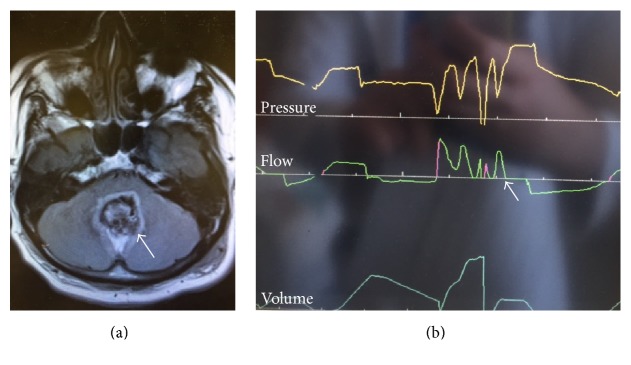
Magnetic resonance imaging of the brain. (a) T2 fluid attenuated inversion recovery (FLAIR) sequence showing medulloblastoma status after resection causing effacement of the fourth ventricle (arrow). (b) Ventilator picture showing cluster breathing pattern (arrow).
